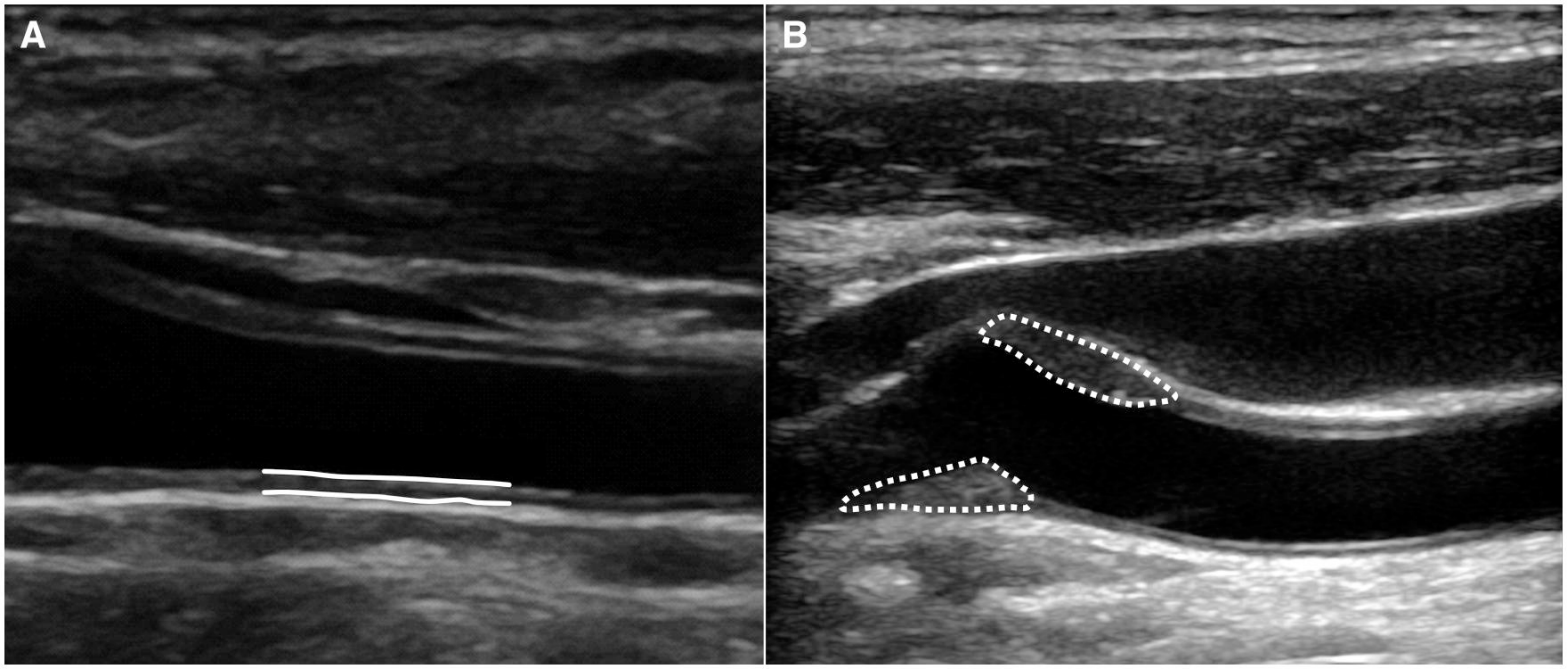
Carotid ultrasound examinations showing measures of carotid intima media thickness (cIMT) and presence of plaque (yes/no). The cIMT was automatically measured in a carotid intima media section with a length of approximately 1 cm. The cIMT was measured in millimetres, the maximum and mean cIMT of all projections (120, 150, 210, 240 degrees) was registered for each neck side, and the mean value of the highest maximum of both sides (right and left side) was registered for each participant. A plaque was registered as present when focal thickening of the cIMT was present, or if the IMT exceeded 1.5 mm, in accordance with the Mannheim consensus. (A) Solid lines outlining a measured section with normal cIMT. (B) Dotted lines indicate plaques.
